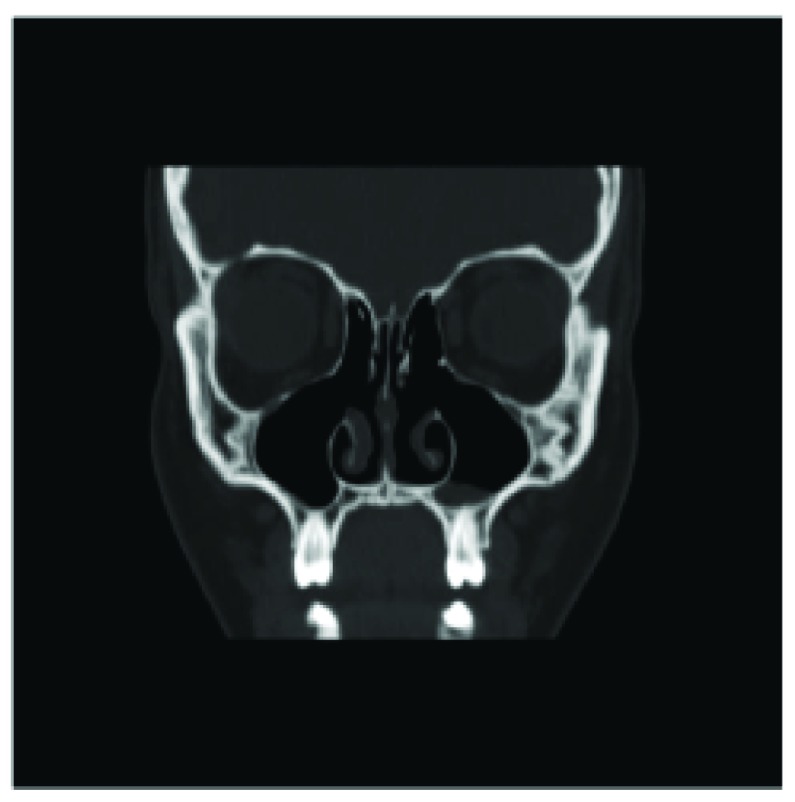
Non-contrasted coronal CT scan from two years prior to patient presentation. Only mild mucosal thickening of the floor of the left maxillary sinus and post-surgical changes related to endoscopic sinus surgery were noted.
